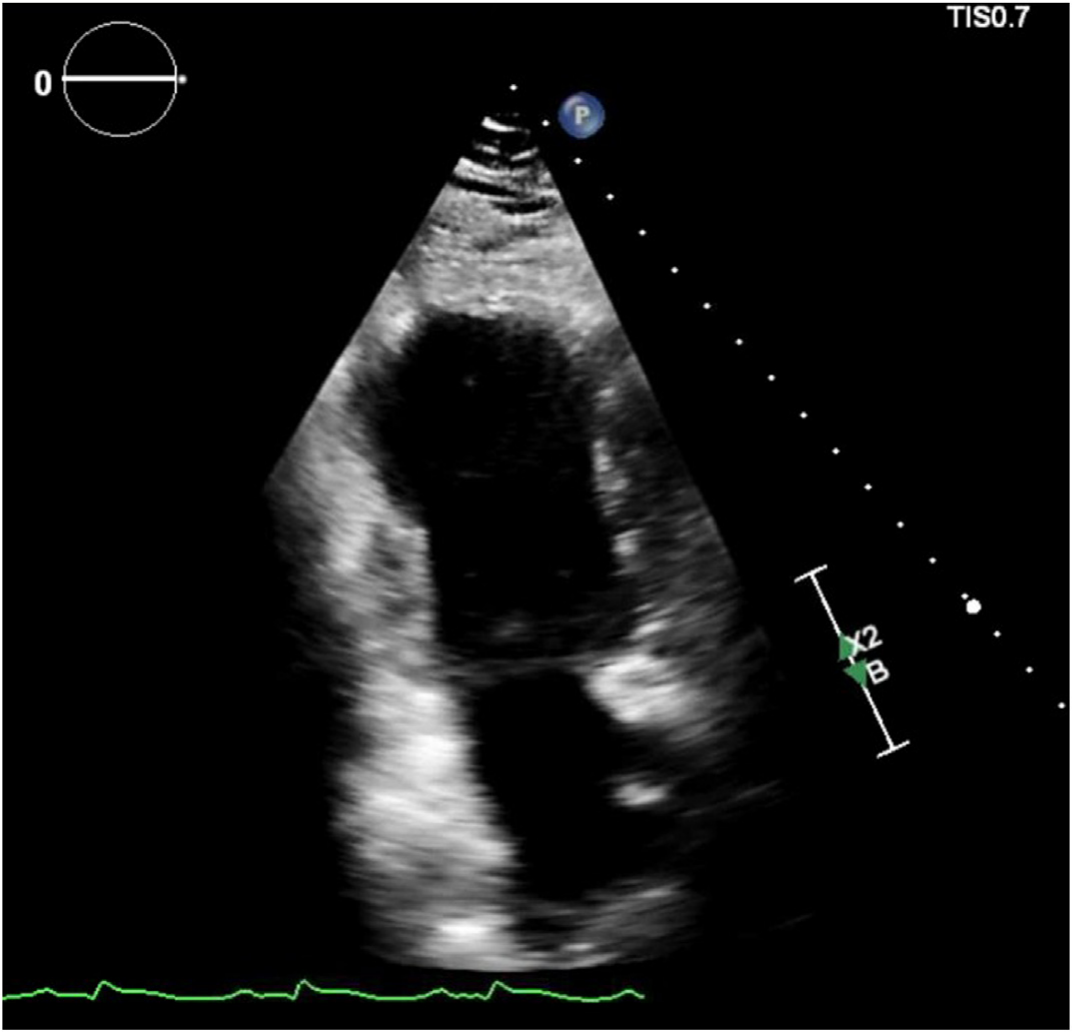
Echocardiogram Showing Apical Ballooning of the Left Ventricle
